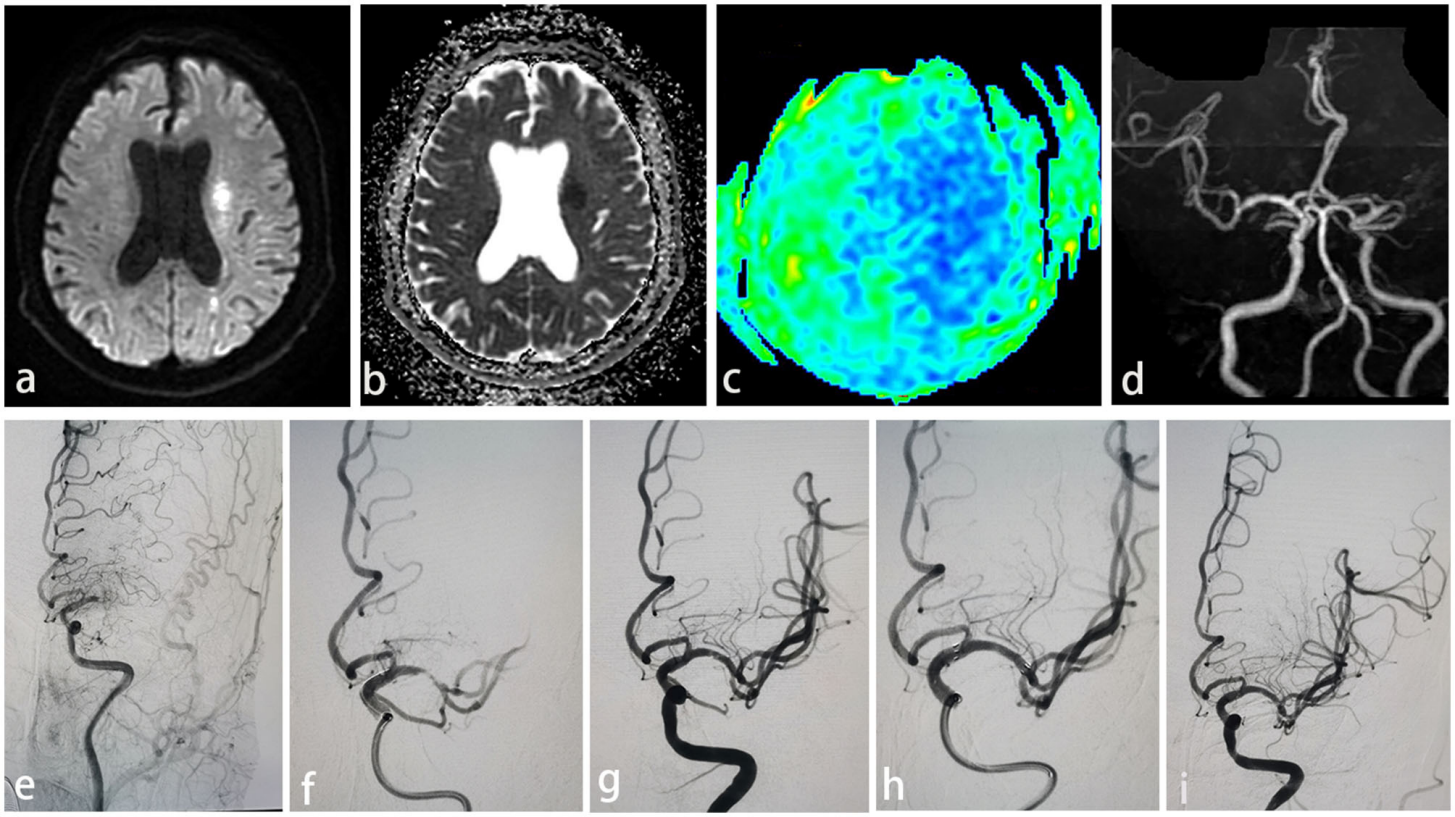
(a) Watershed infarction of left hemisphere in DWI. (b) Watershed infarction of left hemisphere in ADC. (c) Reduced perfusion of left hemisphere. (d) Occlusion of left MCA showing in MRA. (e) Acute left MCA occlusion in DSA. (f) Severe stenosis (>70%) with associated flow limitation after mechanical thrombectomy with a stent retriever. (g) Possible dissection after mechanical thrombectomy. (h) Deploy 3.0 mm × 15 mm Neuroform EZ stent via microcatheter XT-27. (i) Good patency and no in-stent stenosis in 6-months DSA follow-up.
